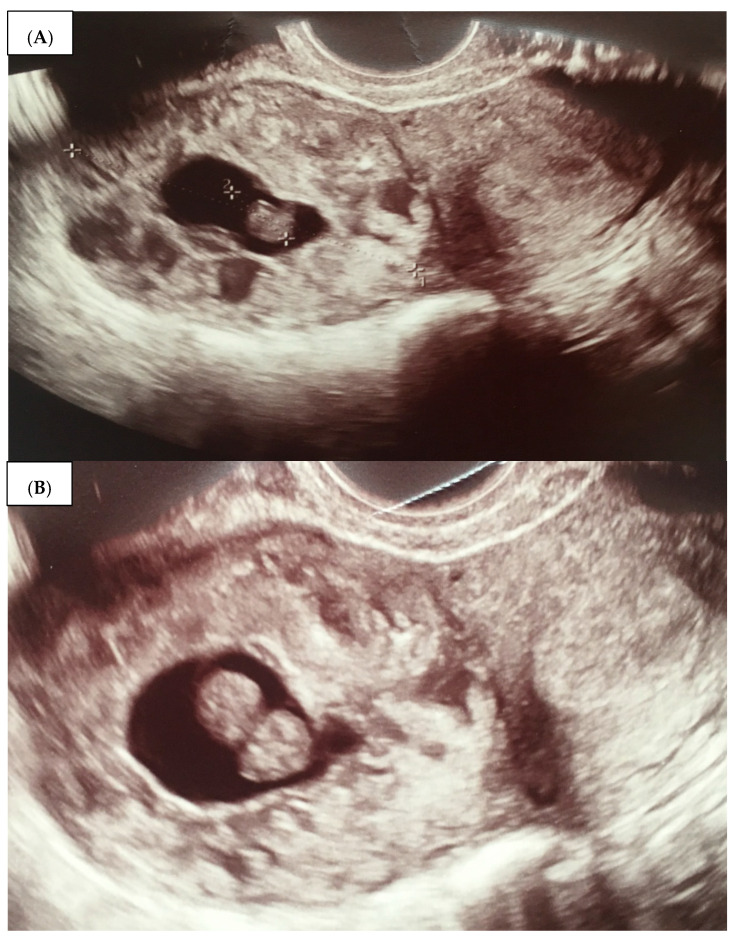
(A,B)—Patient A—a 2D transvaginal ultrasound of an interstitial ectopic pregnancy within the right uterine horn in different dimensions.
